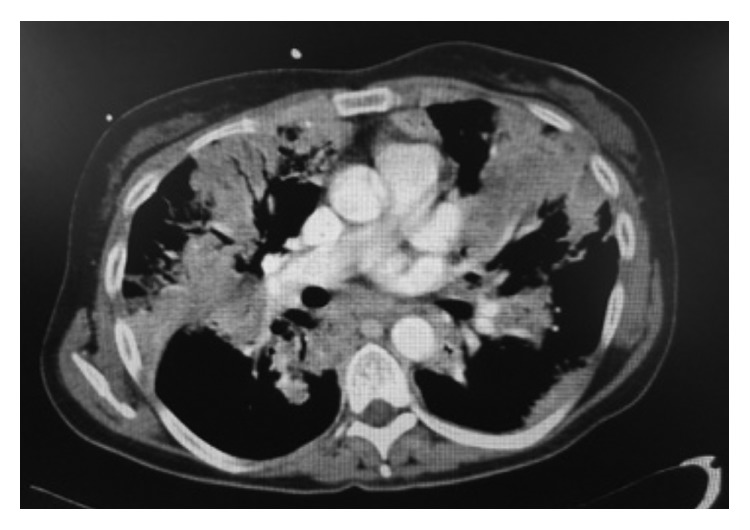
CT-pulmonary angiogram showing extensive multifocal consolidations, reactive mediastinal lymphadenopathy with no pulmonary embolism.
